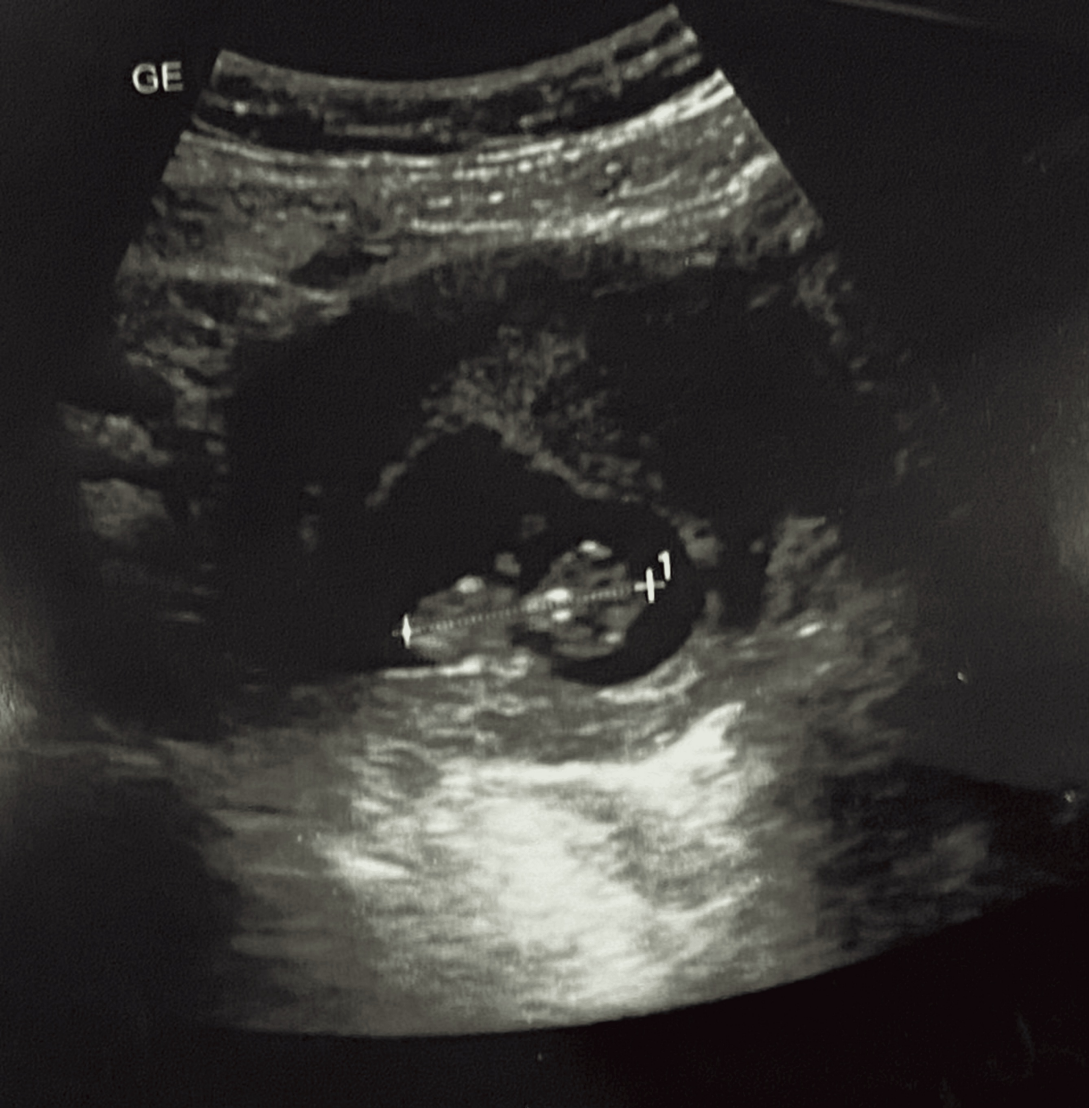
Figure shows early dating obstetric ultrasound scan of the patient at 9 weeks and 6 days period of gestation (POG) which reveals a singleton viable intrauterine pregnancy. Crown-rump-length measurements were 2.99 cm and given a revised estimated due date (REDD). No fetal anomalies were detected.
